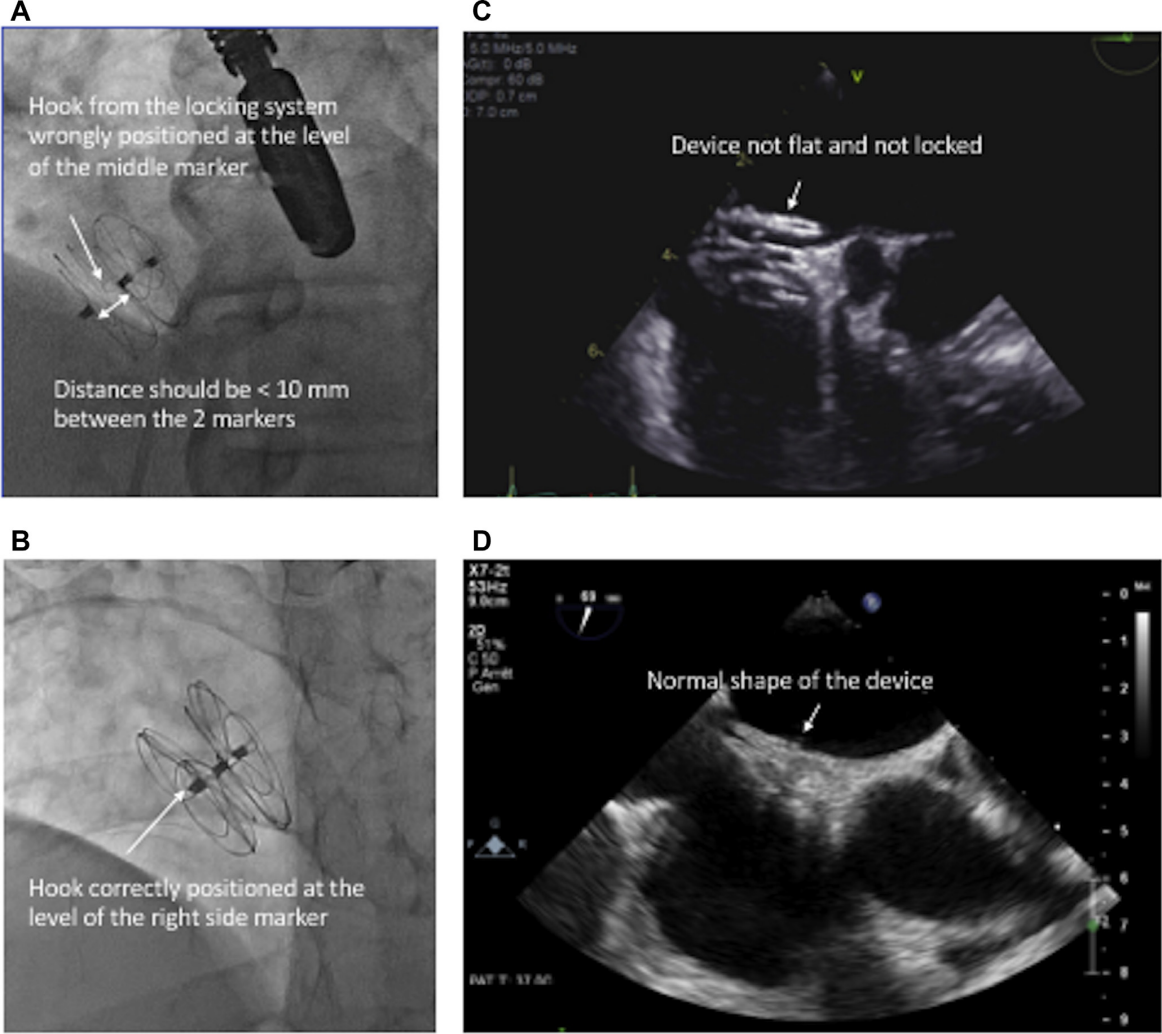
(A) After patent foramen ovale device release, the right disk is not properly locked. The loop of the locking system can be seen at the level of the middle marker (arrow), whereas it should be seen (B) at the level of the marker of the right disk, as shown in a correctly deployed device. Furthermore, the distance between the right atrium marker and the middle marker should be <10 mm ([A] incorrect; [B] correct). (C) Final images of the procedural transesophageal echocardiography showing that the right disk was not flat and therefore properly locked. (D) Transesophageal echocardiography at 6 months showing that the device flattened and took its usual expected shape.
